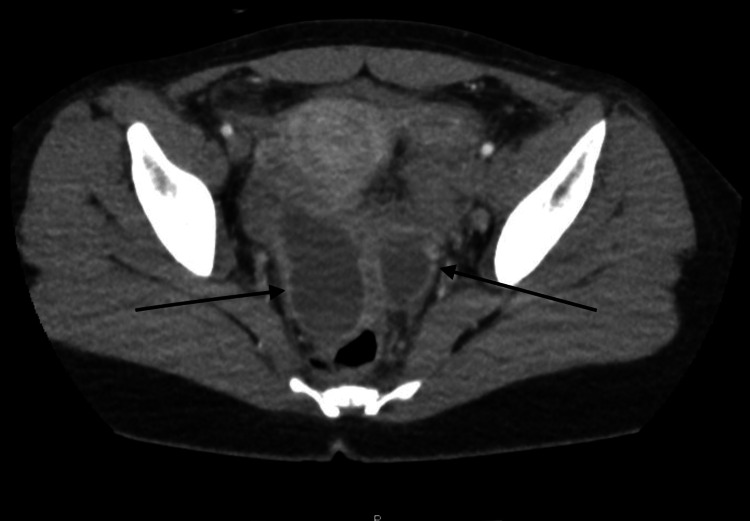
CT abdomen/pelvis (axial plane) The image shows bilateral fluid collections (arrows) suggestive of bilateral tubo-ovarian abscess.
